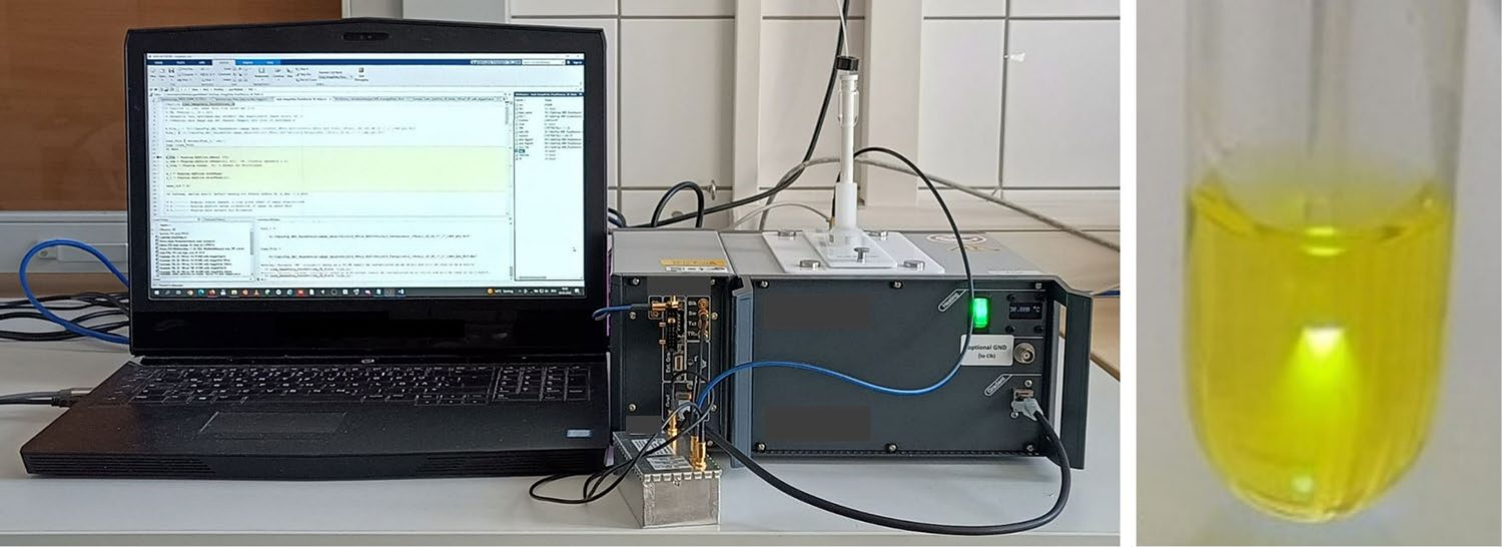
Experimental setup. *Left image*: Tabletop MRI with magnet, controller, small preamplifier and laptop. *Right image*: NMR tube with fiber positioned in pure H_2_O containing FMN and favipiravir.
